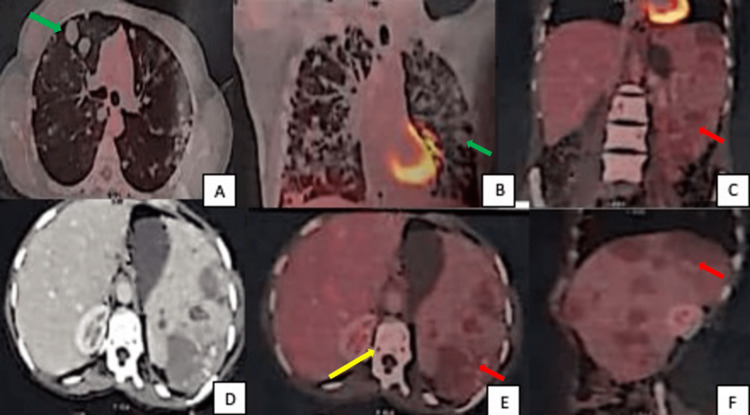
PET images showing lung, skeleton, and splenic lesions Bilateral lung fields (4A, 4B) showing multiple non-fluorodeoxyglucose (non-FDG) avid parenchymal nodules (green arrow) of varying sizes. Splenic lesions (red arrow) show minimal FDG uptake (4C, 4E, 4F). Skeletal lesions (yellow arrow) are also non-FDG avid (4E).
